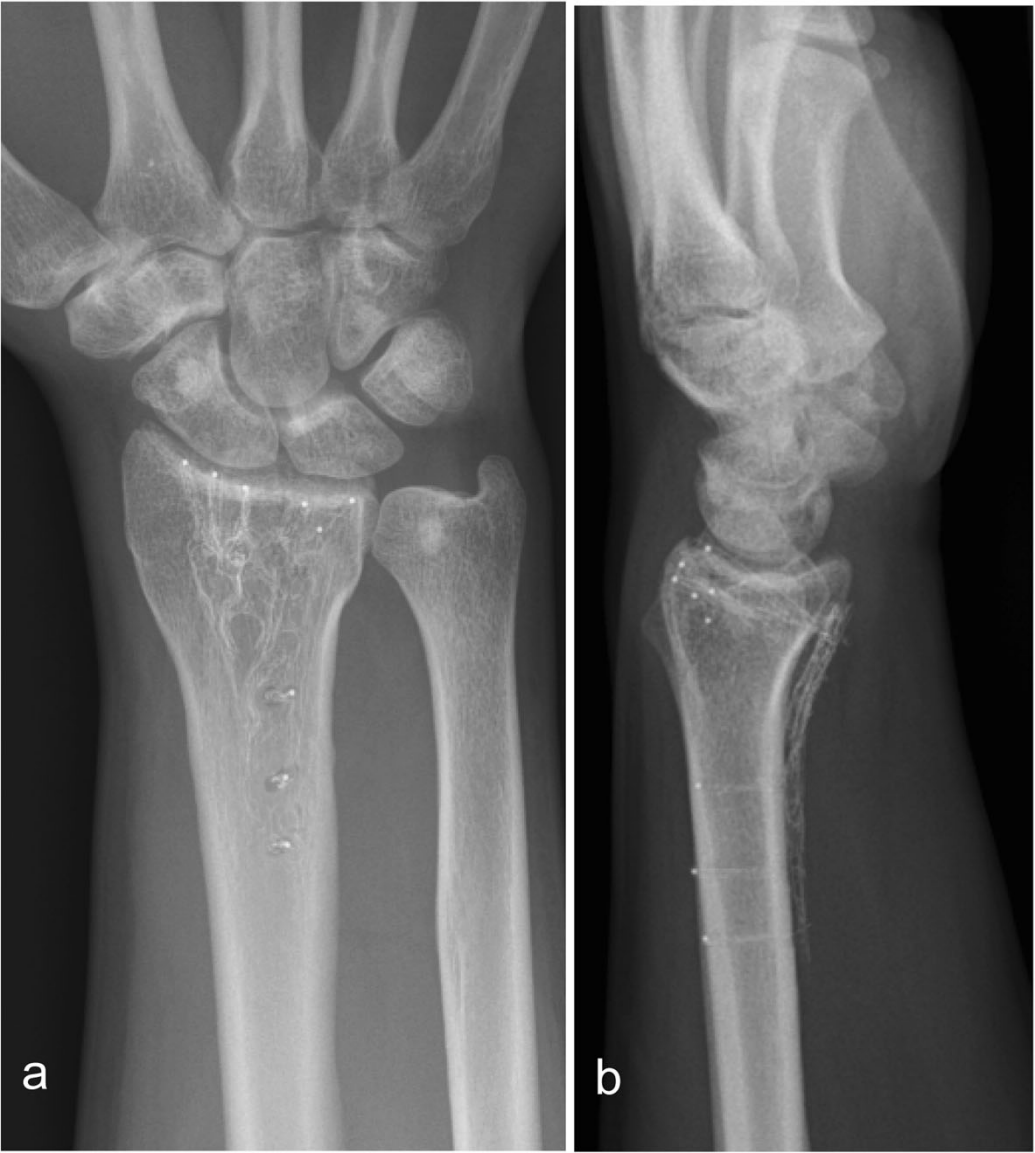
Distal radius fracture treated with a 2.7 mm Carbon/PEEK plate. X-ray 1 year follow up ap (a) and lat view (b)
